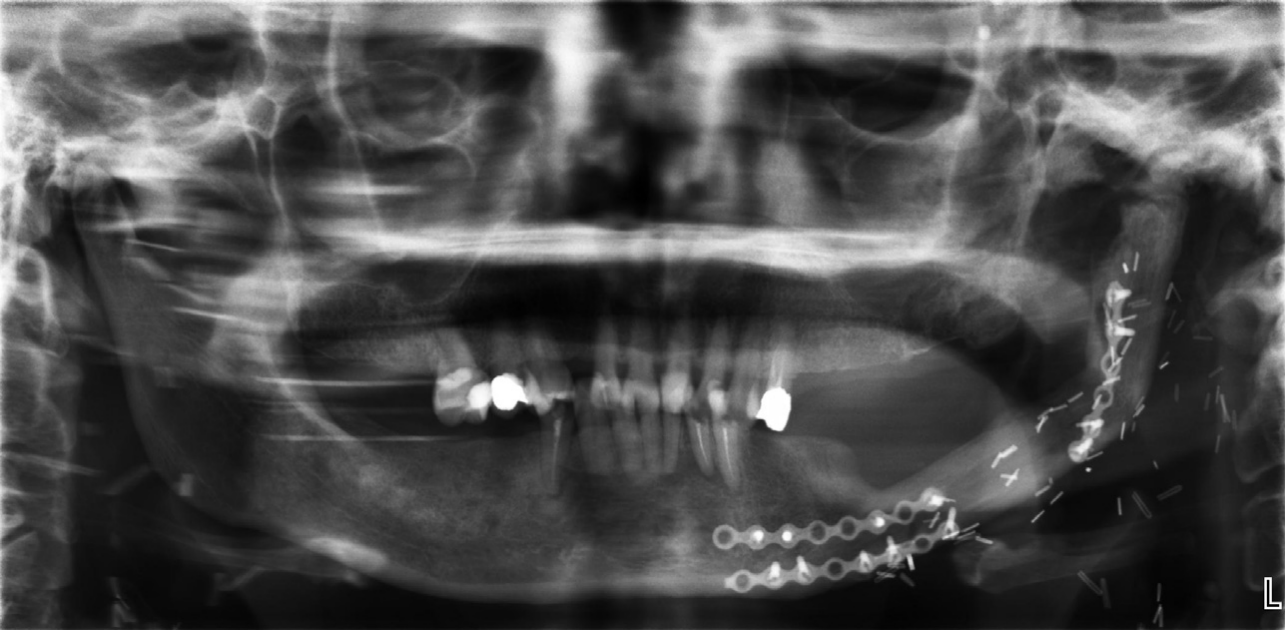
Orthopantomograph shows the marginal mandibulectomy for the left mandible with three plate fixers. *Note*: the pin-like radiopaque structures are actually golden pins used by the villagers as a traditional belief for healing.
